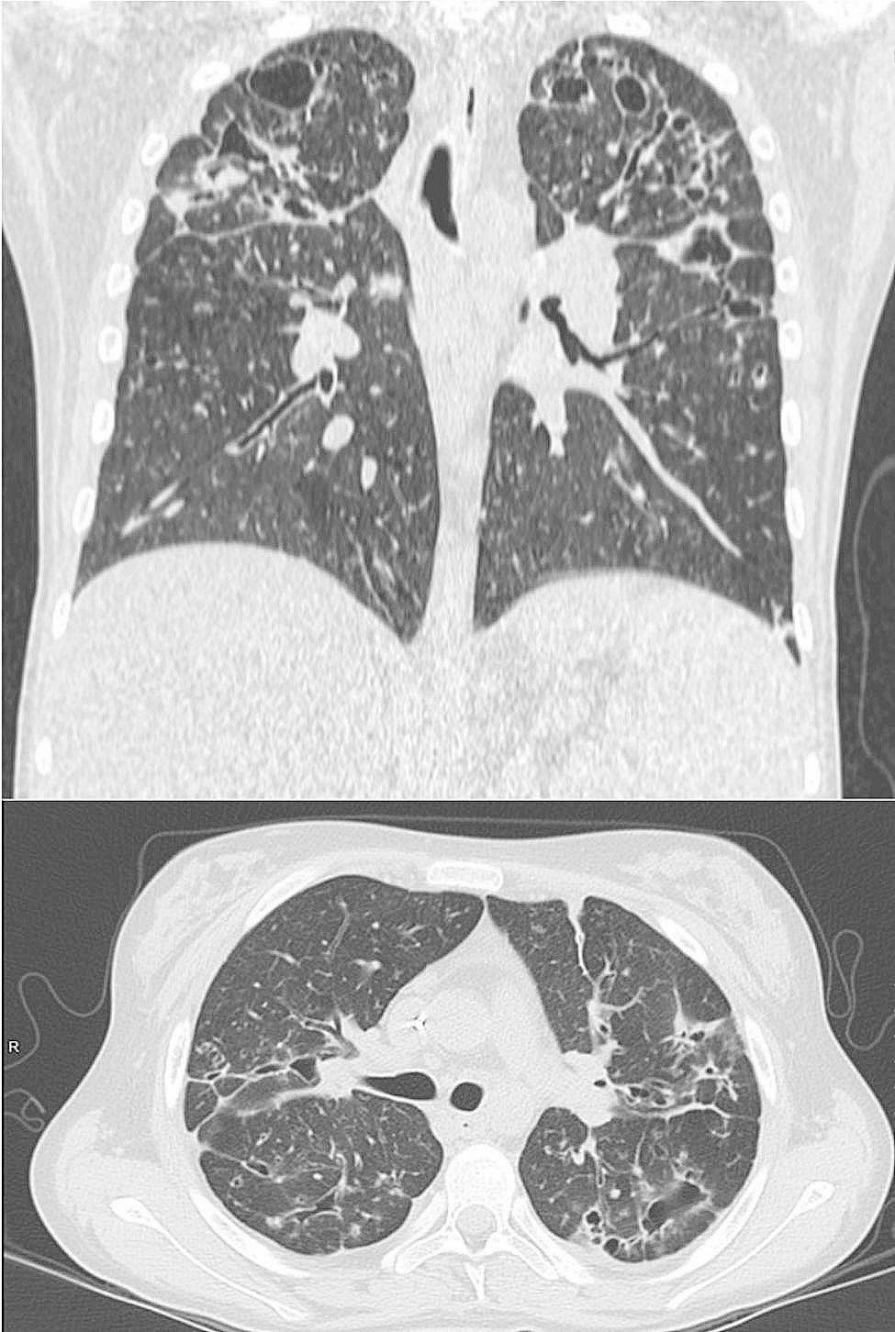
Chest CT-scans show multiple giant apical bronchiectasis and a middle lobe syndrome
